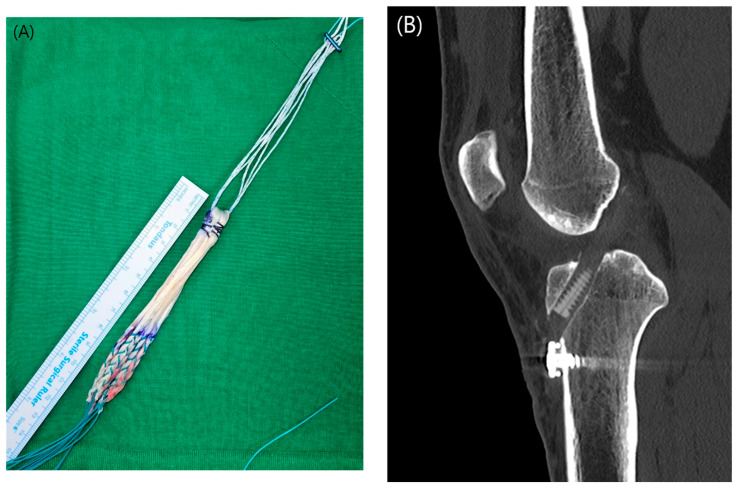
(A) Completed configuration of a four-strand hamstring autograft. (B) Sagittal computed tomography scan depicting the morphology of the full-length tibial tunnel after standard ACL reconstruction.
